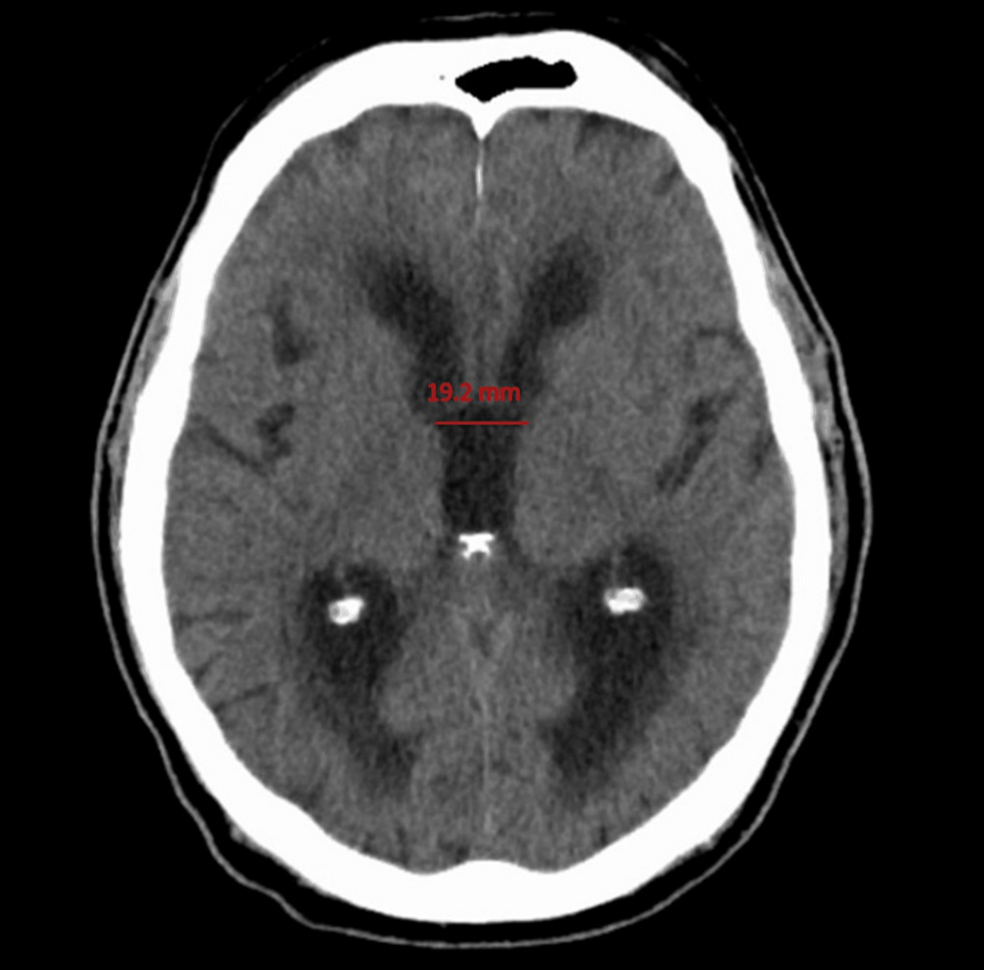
CT of the head without contrast demonstrating increased ventricular size (hydrocephalus) in the lateral and third ventricles.
